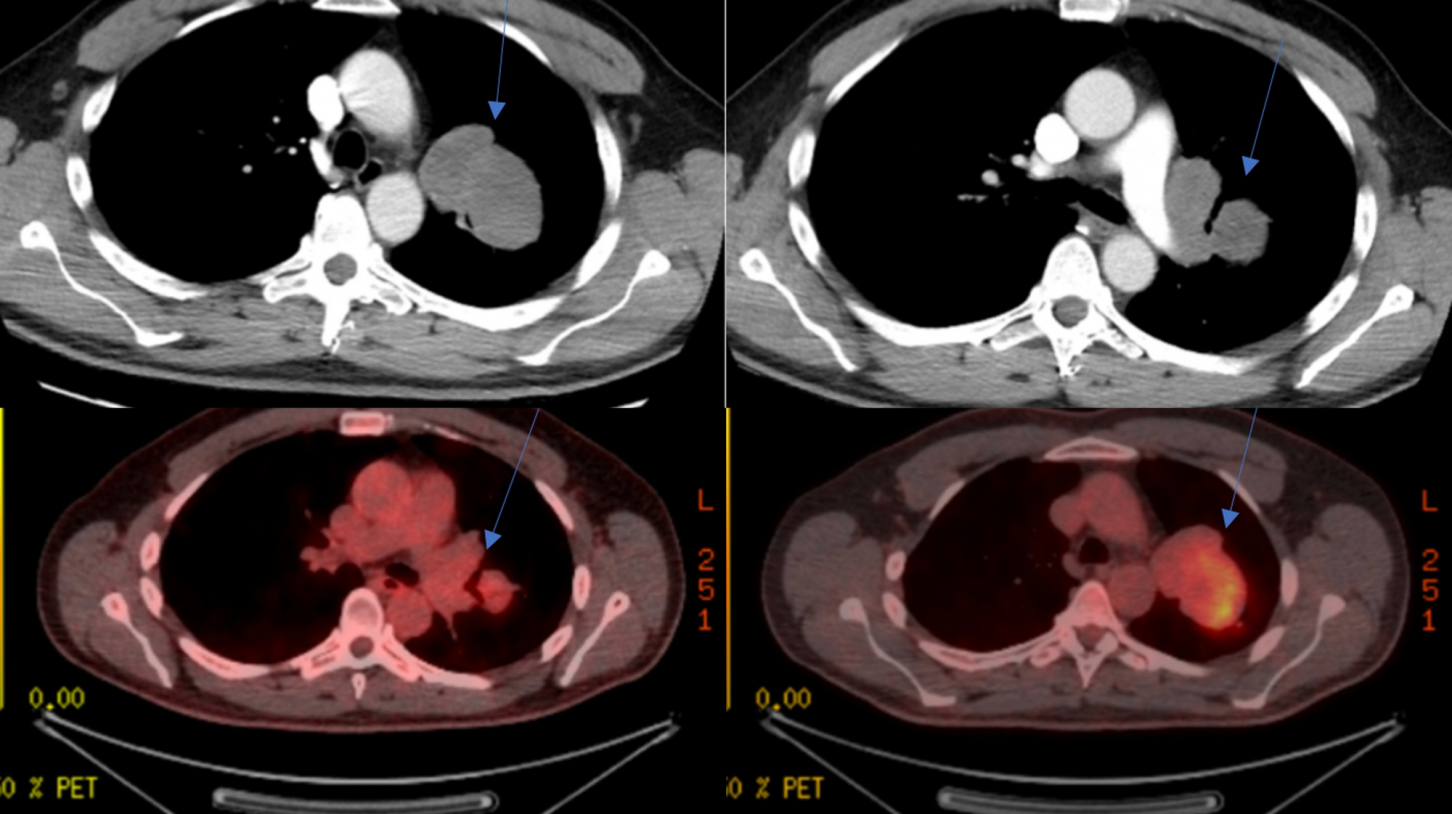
CT/PET scan results The images on the top left and top right show the bilobular, smooth borders of the sarcoma located in the left upper lobe of the lung. The images on the bottom left and bottom right detail the positron emission tomography (PET) scan results showing heterogeneous activity in the area of the mass. The blue arrows indicate the area of interest.
